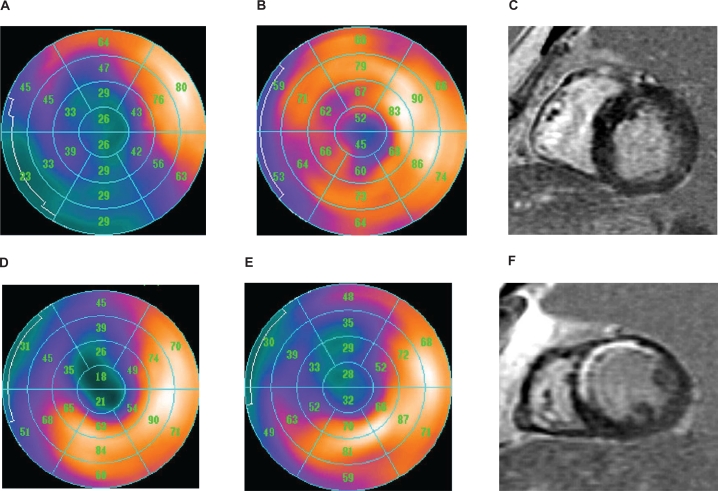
Upper panel: a discordant study with inferior scar identified with MIBI, but viable myocardium with FDG and CMR (A–C). Lower panel: example of a concordant study with anterior scar identified with all three imaging modalities (D–F).
